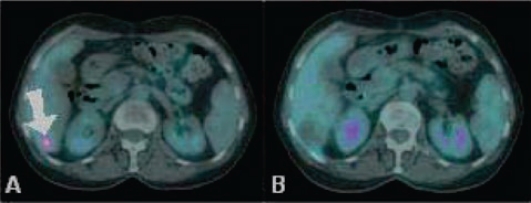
(a) CT/PET before percutaneous thermal ablation of hepatic metastasis from colorectal cancer—FDG uptake by the lesion (arrow); (b) CT/PET performed six months later percutaneous treatment, clearly shows the absence of activity by the lesion (no FDG uptake)
